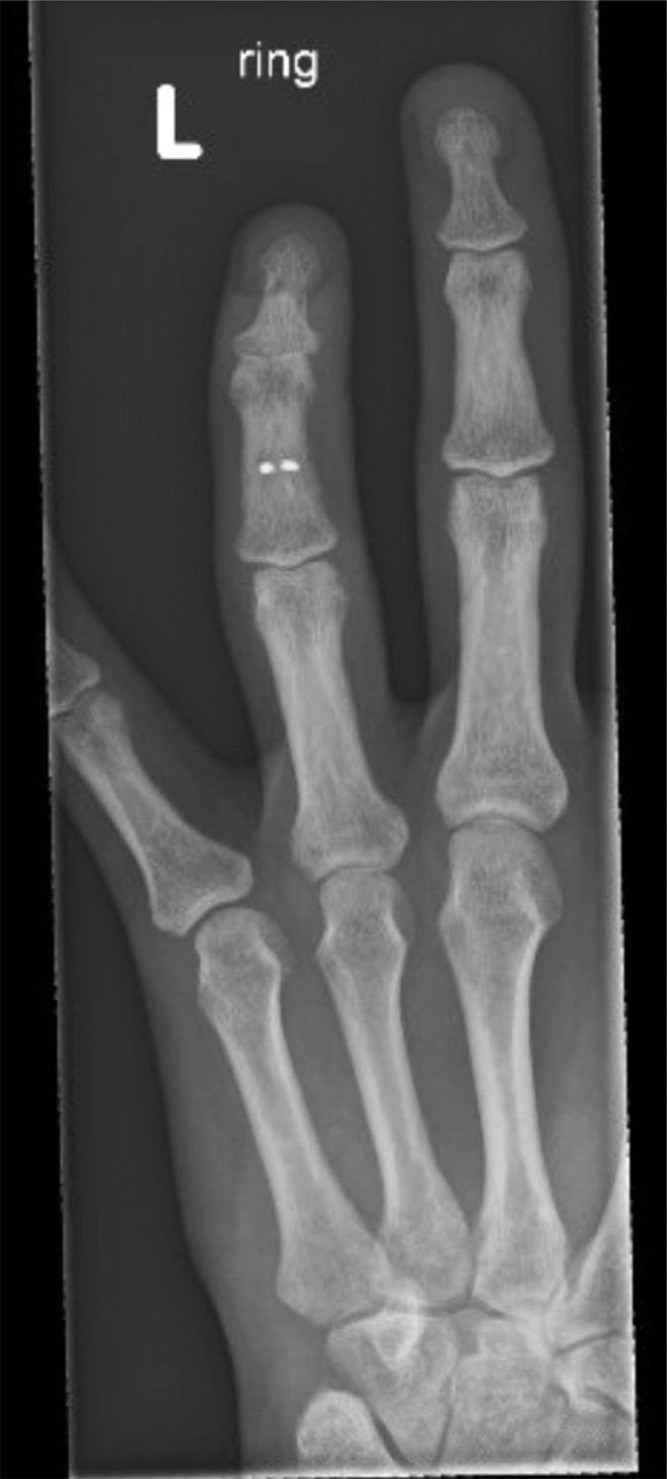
Frontal X-Ray of right hand (Case 2: Post-op).
